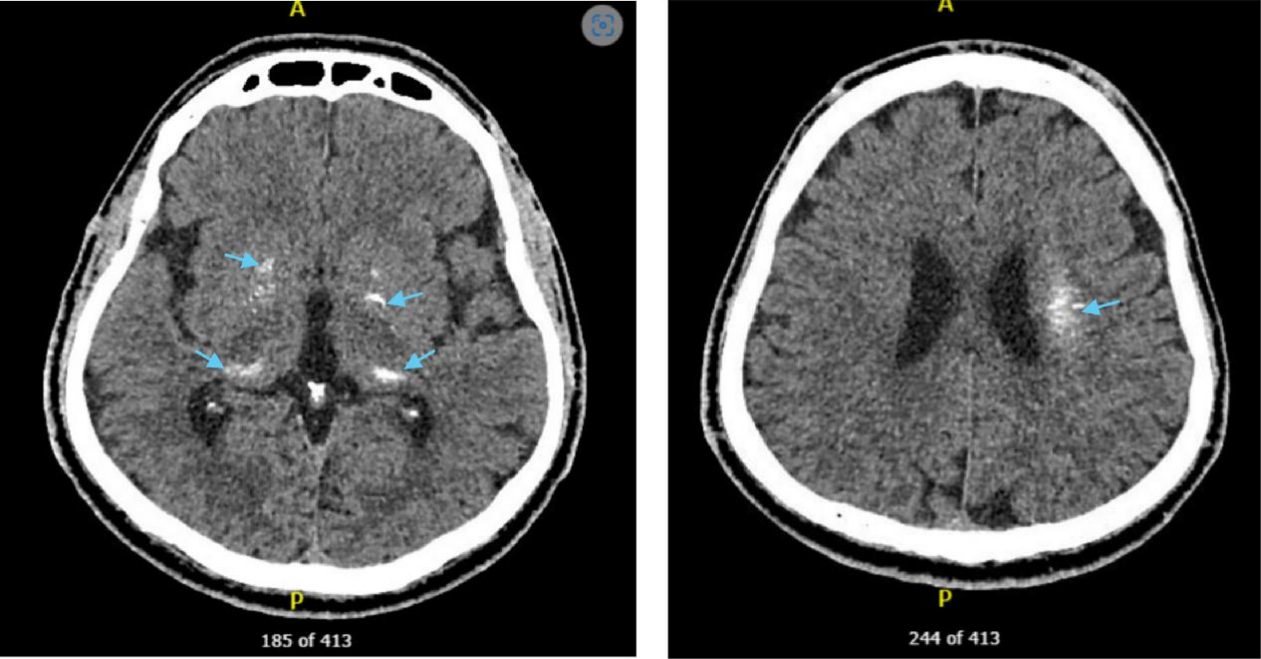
Noncontrast axial computed tomography images of the head in 2022 showed calcifications in the bilateral basal ganglia, left centrum semiovale, and dentate nuclei of the cerebellum.
